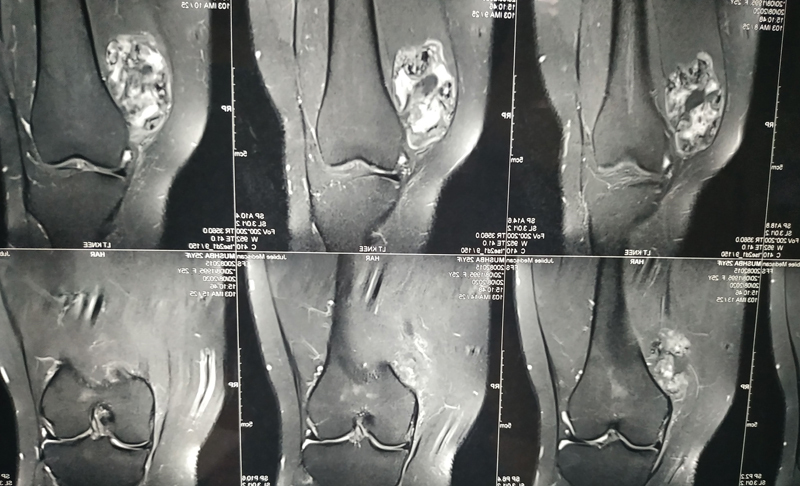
An MRI scan of the patient showing a large soft-tissue mass measuring 6.6 cm × 5 cm × 3.7cm arising from the vastus medialis muscle in the anterior compartment of the left thigh. The lesion had areas with signal intensity similar to that of subcutaneous fat with soft-tissue nodules. There were areas of dystrophic calcification within the lesion. The joint cavity and synovium were unaffected.
